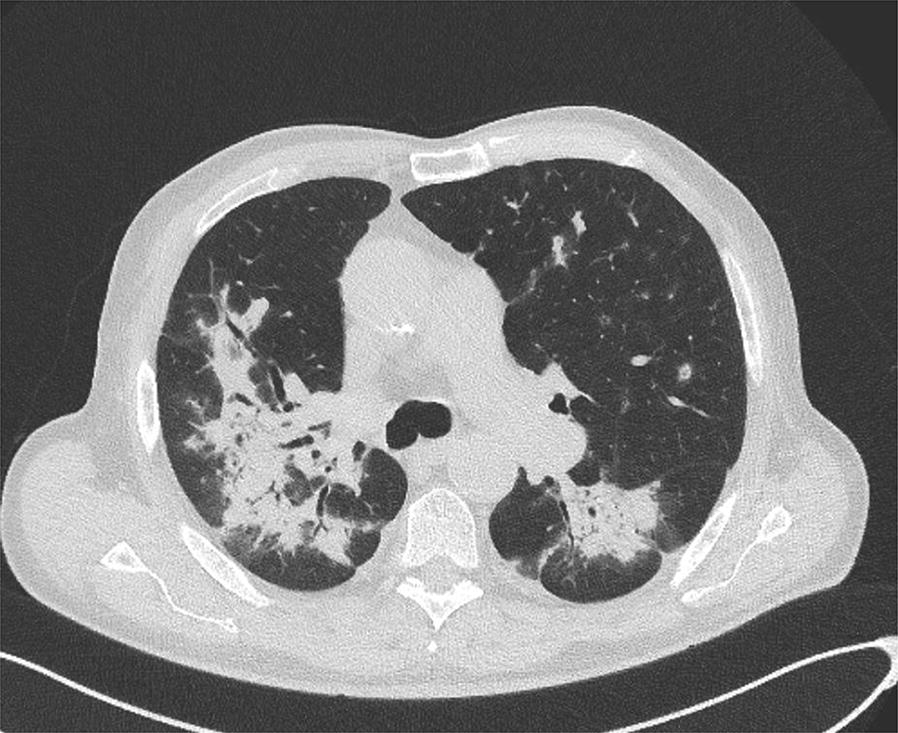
Example of thoracic CT in immune-related pneumonitis. The patient had been treated for NSCLC with pembrolizumab for 2 months. He developed acute respiratory failure. CT showed the typical organizing pneumonia pattern
